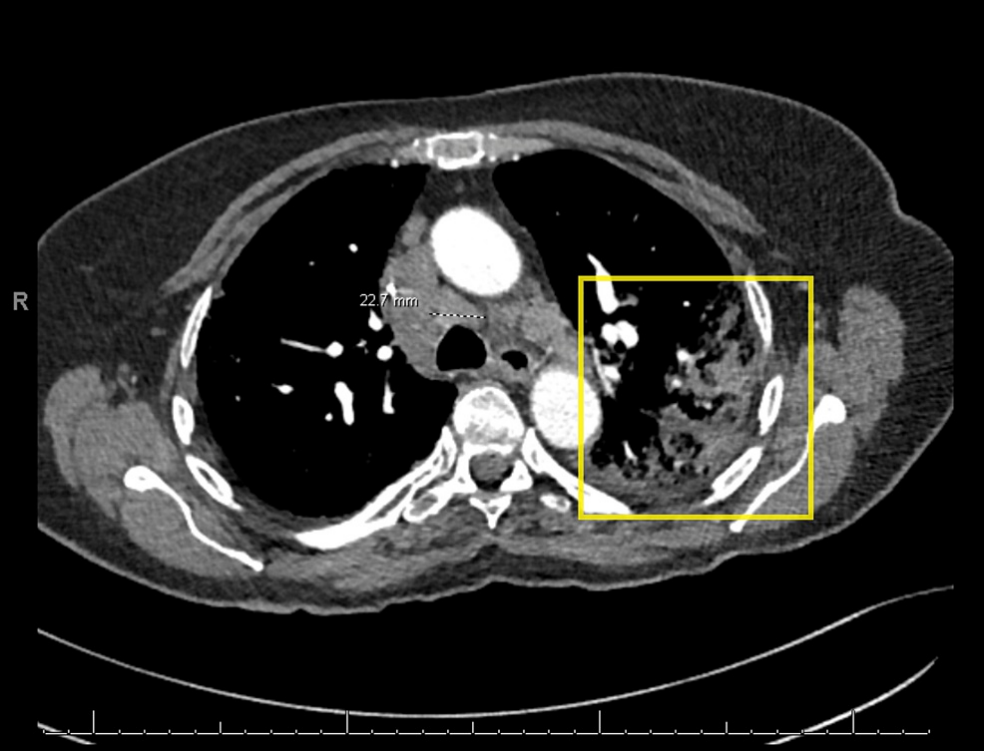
Computed tomography of the chest with contrast showing left lung infiltrates (yellow box) and mediastinal adenopathy (dotted black and white line)
